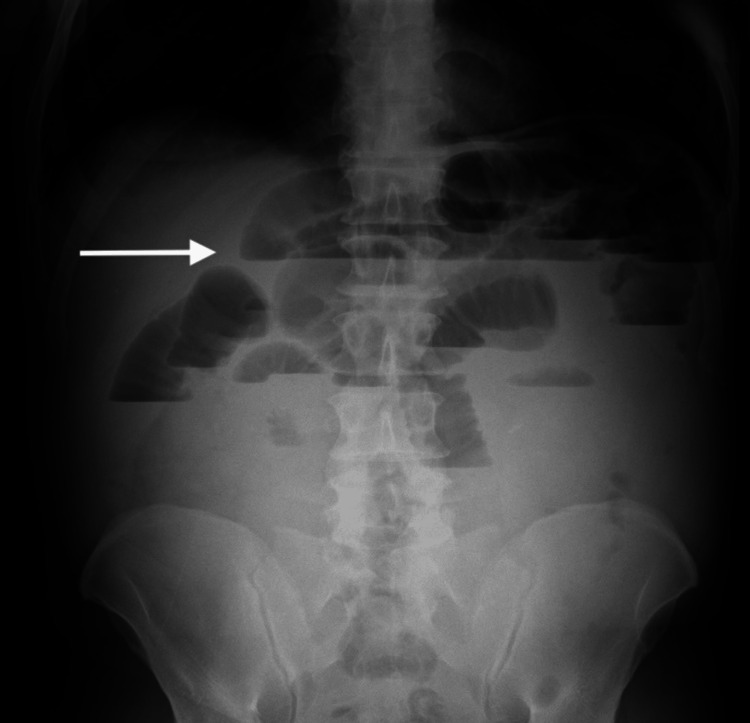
Preoperative abdominal X-ray The arrow points to air-fluid levels on distended bowel loops consistent with small bowel obstruction
